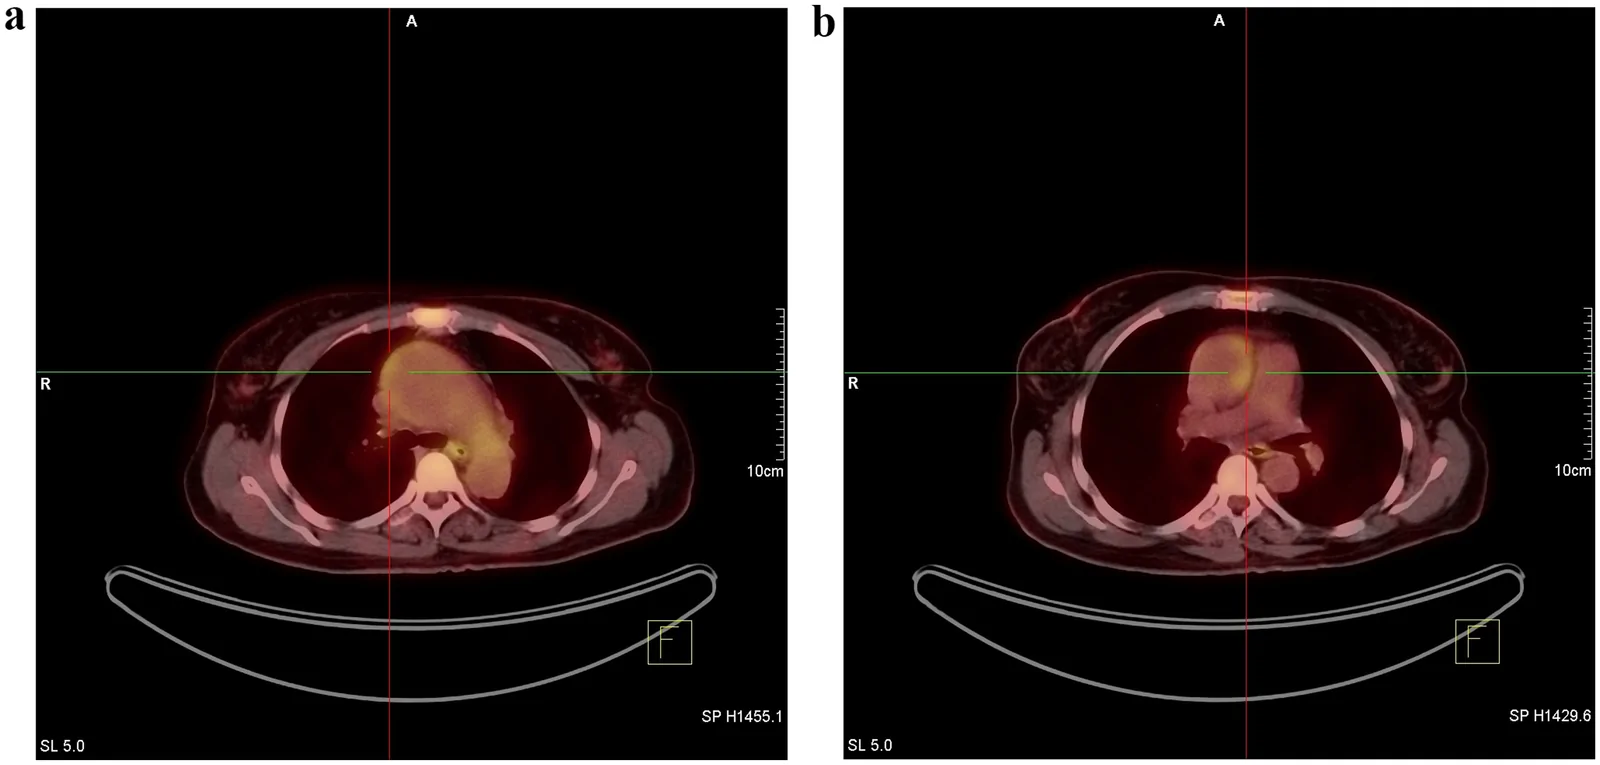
a, b Axial ¹⁸F-FDG PET/CT fusion image demonstrating FDG avidity along the lateral wall of the left ventricle, consistent with myocardial involvement in the context of active Takayasu arteritis
